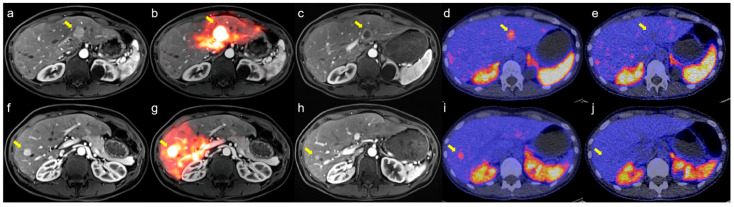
Representative case demonstrating treatment response after sequential bilobar Y-90 SIRT for neuroendocrine liver metastases (NELM). (a,f) Baseline contrast-enhanced MRI before SIRT showing arterial-enhancing metastases in segment 2 (a, arrow) and segment 8 (f, arrow); (d,i) Baseline [^68^Ga]Ga-DOTATOC PET/CT showing intense somatostatin receptor (SSTR) uptake corresponding to the MRI-visible lesions (arrows); (b,g) The patient subsequently underwent sequential bilobar Y-90 SIRT, with arrows indicating the treated lesions; (c,h) Four-month follow-up MRI demonstrates reduction in lesion size and decreased arterial enhancement in the treated metastases (arrows), consistent with treatment response; (e,j) Follow-up [^68^Ga]Ga-DOTATOC PET/CT shows markedly reduced SSTR uptake in treated lesions (arrows), with no evidence of new SSTR-avid disease.
